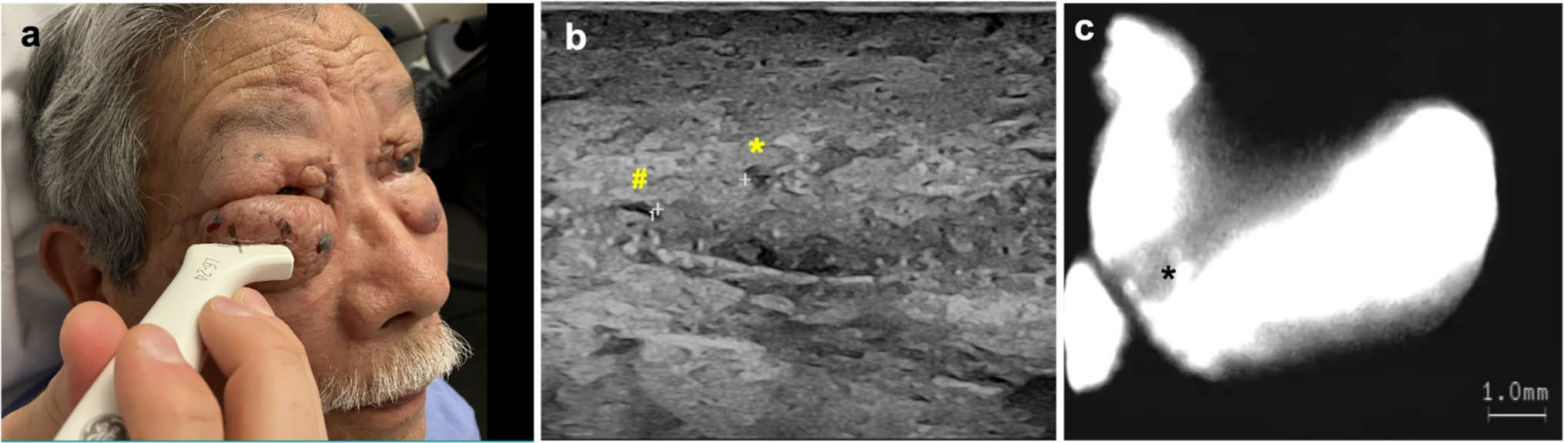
a Preoperative high frequency ultrasound (24 MHz) to localize lymphatic vessels and veins in the edematous eyelid tissue, b ultrasound image showing a compressible vein (yellow hash) 3 mm next to a lymphatic vessel (yellow asterisk), c ICG imaging identifying lymphatic collector
